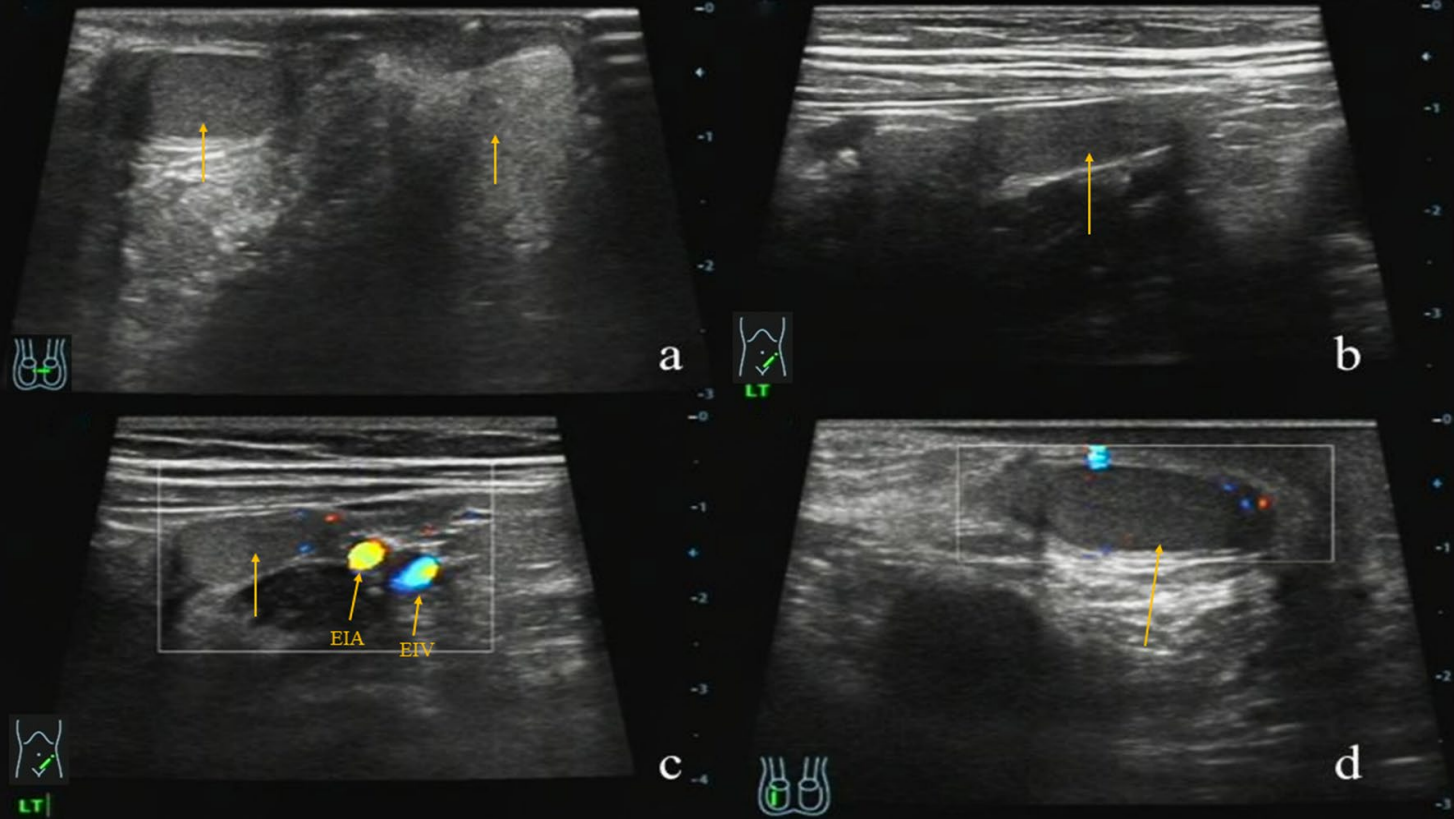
Ultrasound features of IAT on the left side of an 8-months-old boy. (a) The cross section of the scrotum showed that the left testicular was not in the left scrotum, and the right testicular was in the right scrotum (b) and the left testicular was showed next to the left external iliac artery (EIA) in the abdominal cavity (c) the left testis without obvious movement, CDFI showed punctate blood flow signal in the parenchyma (d) and the longitudinal section and the CDFI showed that the right testis was normal (arrows).
